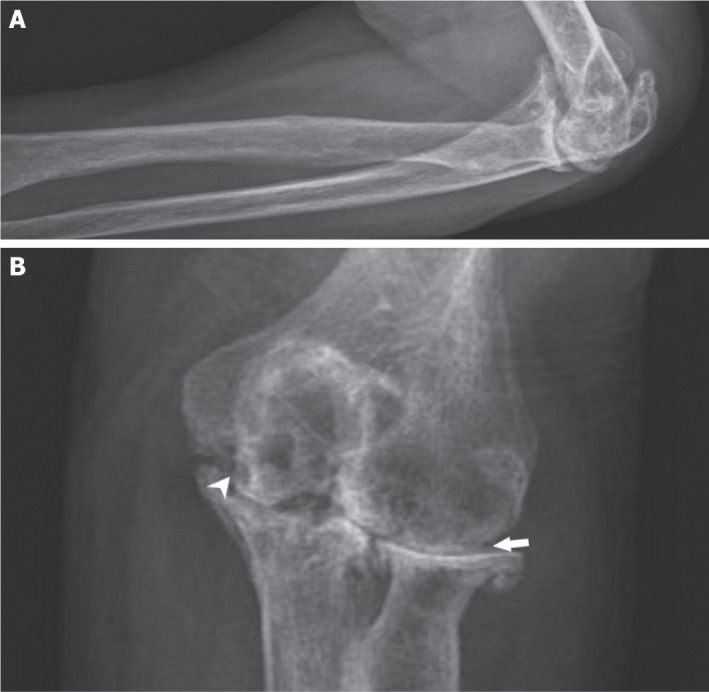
(A) Lateral and (B) AP X-ray of the left elbow, showing juxta-articular osteoporosis, erosion (arrowhead) and narrowing of the joint space (arrow).
